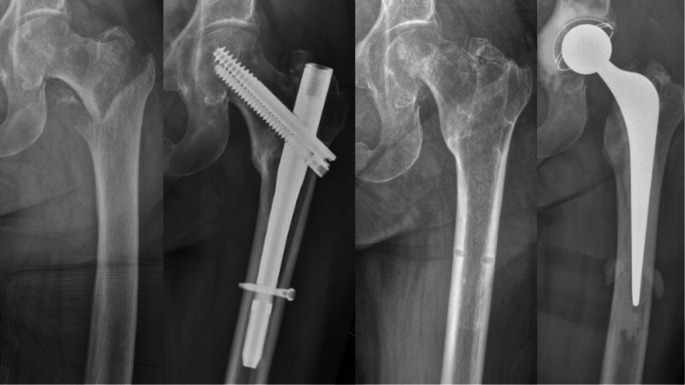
Trochanteric hip fracture treated with a short Trigen nail. The implant was removed 25 months after fixation due to lateral hip pain. Within one week postoperatively, the patient developed groin pain and an inability to bear weight. Radiographs obtained seven days after extraction revealed a femoral neck fracture, subsequently managed with total hip arthroplasty
